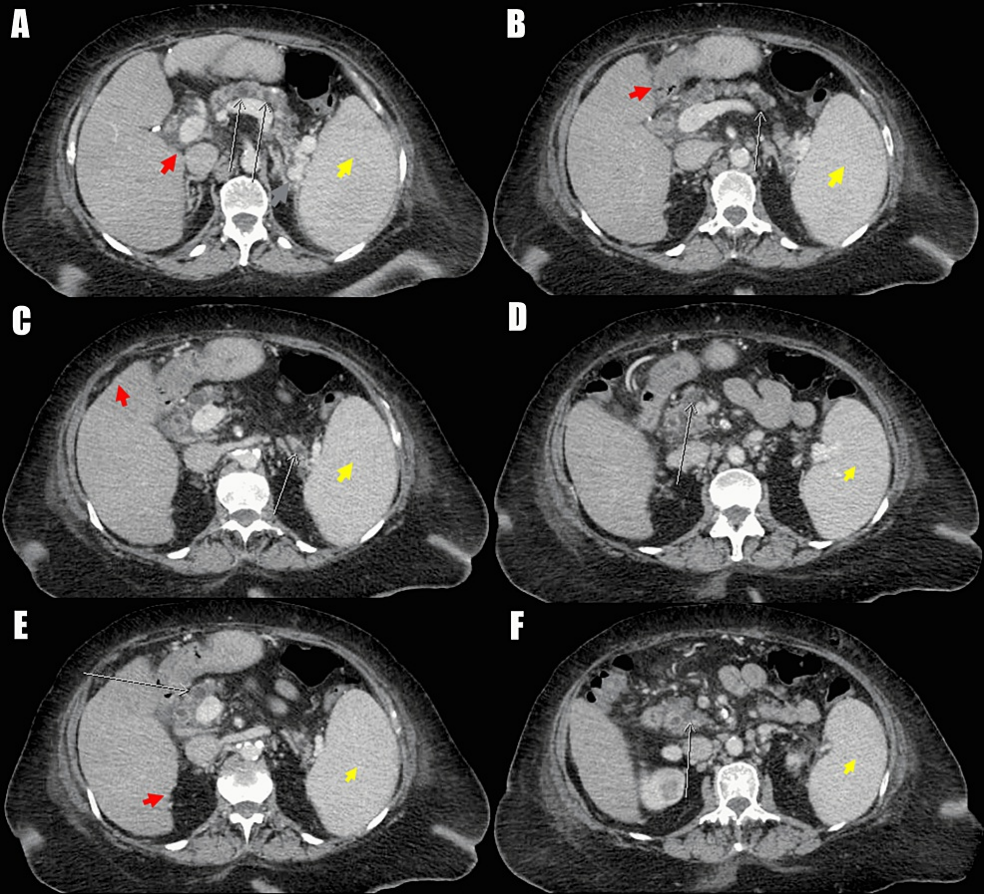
Computed tomography of the abdomen: transverse views. A: White arrows demonstrating intraductal papillary mucinous neoplasms within the pancreas. Red arrow demonstrating cirrhotic liver with collateral vessels and nodular border. Blue arrow demonstrating portal hypertensive collateral vessels. Yellow arrow demonstrating splenomegaly. B: White arrow demonstrating intraductal papillary mucinous neoplasms within the pancreas. Red arrow demonstrating cirrhotic liver with collateral vessels and nodular border. Yellow arrow demonstrating splenomegaly. C: White arrow demonstrating acute pancreatitis of the pancreatic tail. Red arrow demonstrating cirrhotic liver with collateral vessels and nodular border. Yellow arrow demonstrating splenomegaly. D: White arrow demonstrating intraductal papillary mucinous neoplasms within the pancreas. Yellow arrow demonstrating splenomegaly. E: White arrow demonstrating intraductal papillary mucinous neoplasms within the pancreas. Red arrow demonstrating cirrhotic liver with collateral vessels and nodular border. Yellow arrow demonstrating splenomegaly. F: White arrow demonstrating intraductal papillary mucinous neoplasms within the pancreas. Yellow arrow demonstrating splenomegaly.
